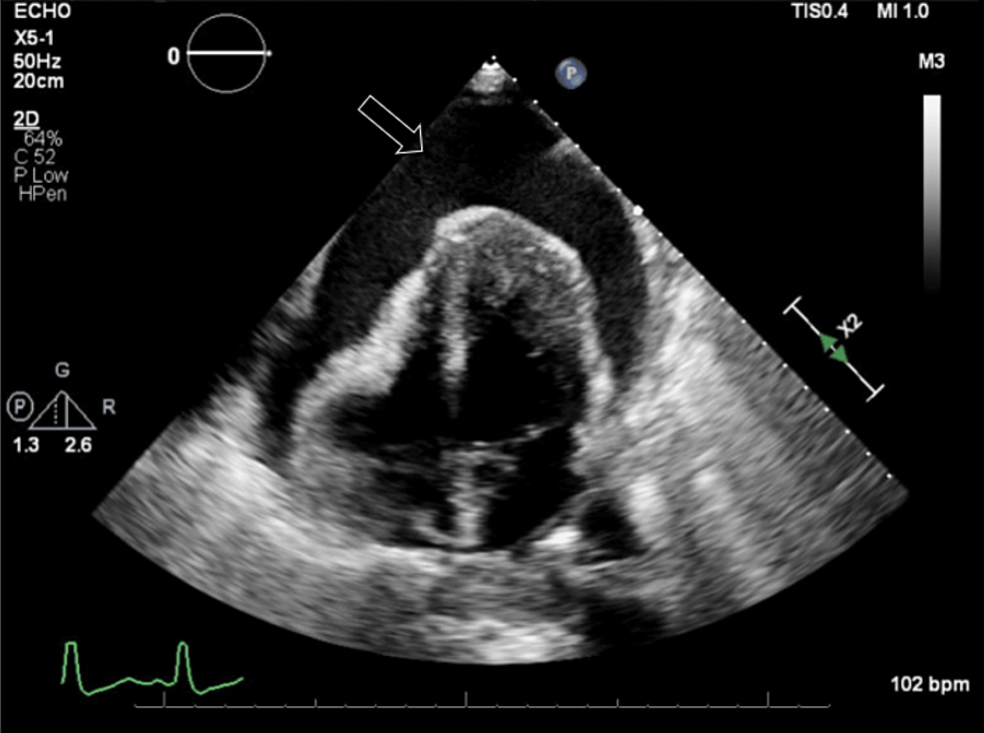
Large pericardial effusion visualized on echocardiography
